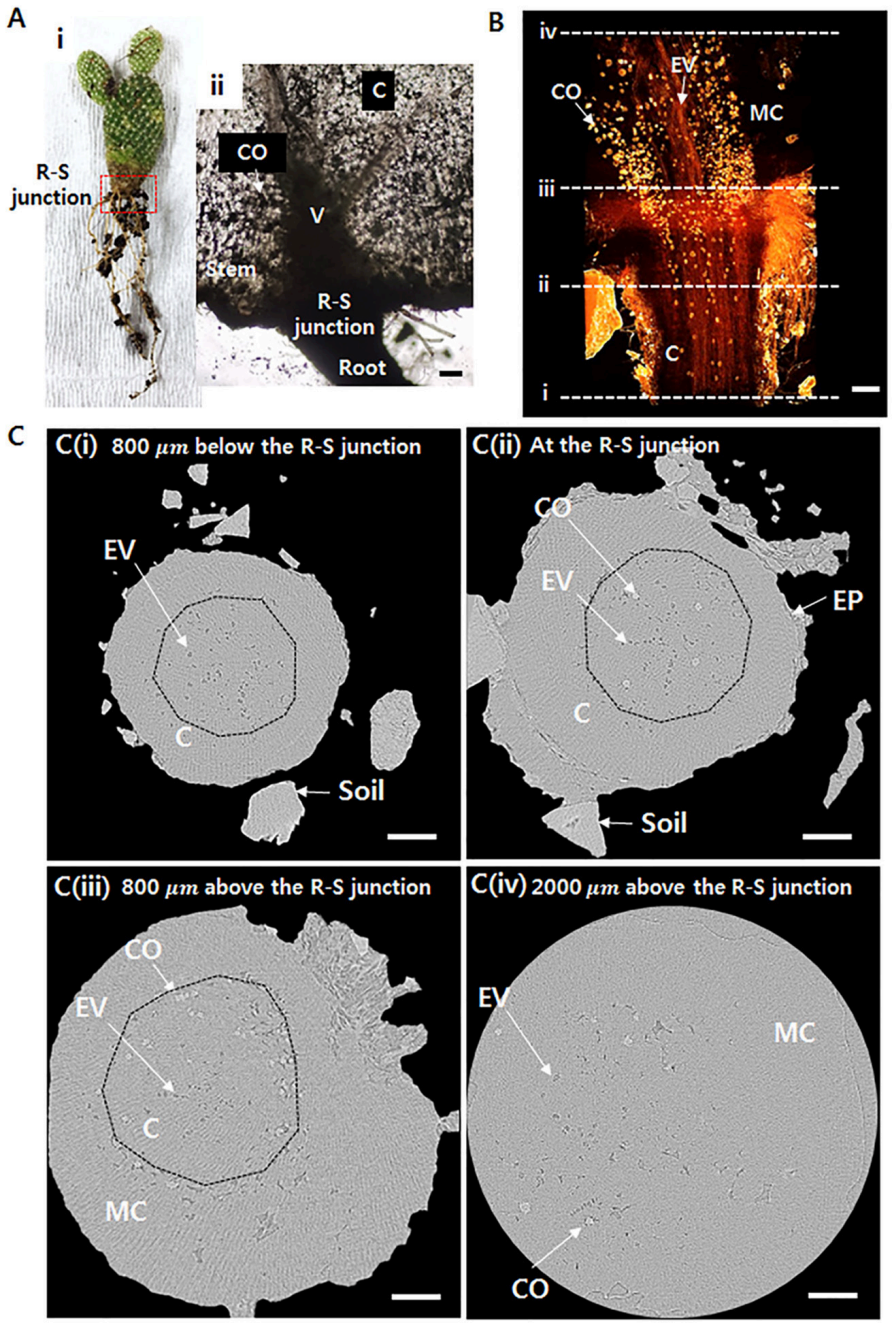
Morphological structures around the root–stem (R–S) junction of *Opuntia microdasys*. (Ai) Optical image of *O. microdasys*. Red dotted box indicates the region of the R–S junction. (Aii) Optical image of the R–S junction of a sliced *O. microdasys* root. (B) 3D image of the *O. microdasys* root visualized by X-ray bioimaging technique. (C) Transverse microcomputed tomography (μ-CT) images (Ci) at 800 μm below the R–S junction, (Cii) at the R–S junction, (Ciii) at the stem 800 μm above the R–S junction, and (Civ) at the stem 2,000 μm above the R–S junction. These (Ci–iv) locations correspond to the dotted lines in (B). R, root; S, stem; CO, calcium oxalate; C, cortex; V, vessel; EV, embolized vessel; EP, epidermis; MC, mucilage cell; dotted lines: endodermis. Scale bar: 200 μm.
